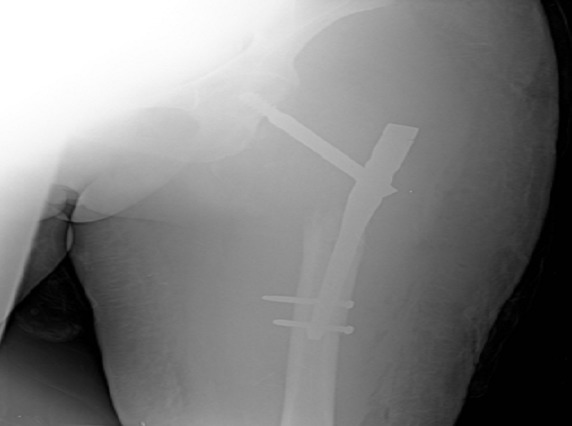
Radiography of the left thigh from the front after an osteosynthesis with a gamma nail
